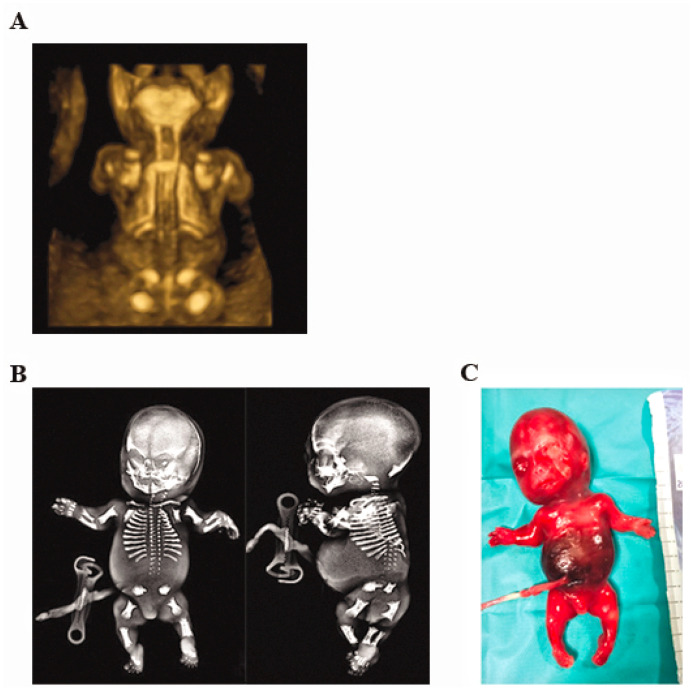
(A) Three-dimensional ultrasound image of the fetus showing reduced ossification of the sacrum, iliac spines, and arched femurs. (B) Radiograph of the fetus showing reduced ossification of the sacrum, iliac spines, and arched femurs. (C) Frontal view of the fetus postmortem.
